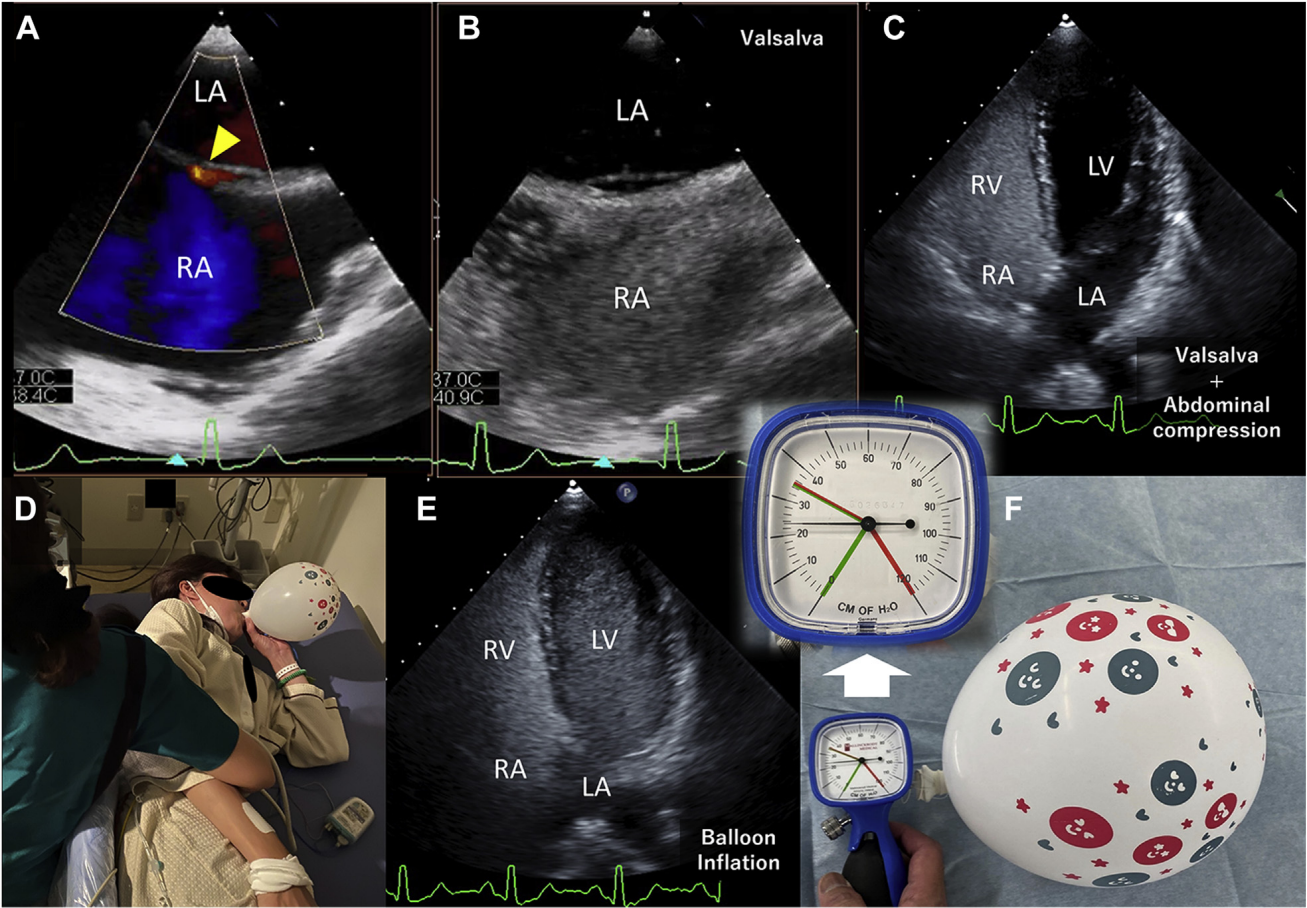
Transesophageal Echocardiography and Transthoracic Echocardiography Images, Party Balloon Inflation Maneuver, and Pressure Measurement Color Doppler transesophageal echocardiography images with an omniplane angle of 120° (A) and saline contrast transesophageal echocardiography with an omniplane angle of 120° (B) during spontaneous Valsalva maneuver. Saline contrast transthoracic echocardiography images during spontaneous and abdominal compression Valsalva maneuver (C) and balloon inflation maneuver (E). Patient performs balloon inflation maneuver (D). Pressure measurement in the dry laboratory (F). The white arrow shows an enlarged view of the face of the cuff manometer during balloon inflation. LA = left atrium; LV = left ventricle; RA = right atrium; RV = right left ventricle.
